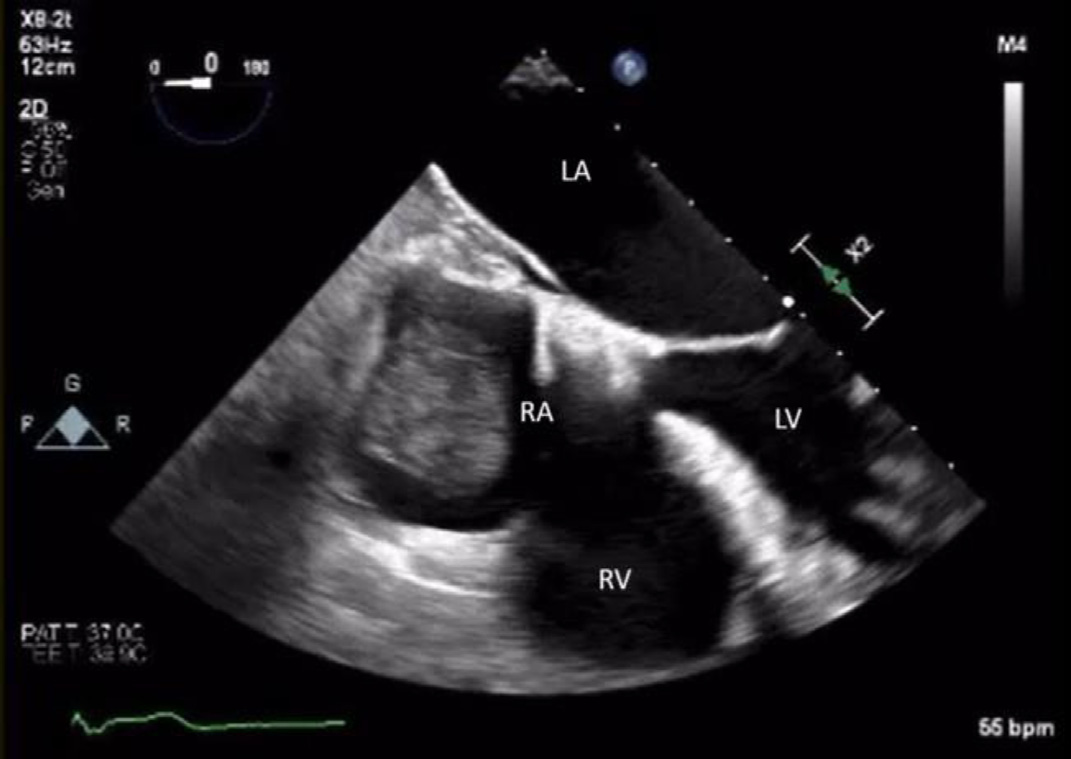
Transesophageal Echocardiography Showing the Residual Stalk of the Mass Attached to the Superior Vena Cava–Right Atrial Junction 3.3 Years Later LA = left atrium; LV = left ventricle; RA = right atrium; RV = right ventricle.
